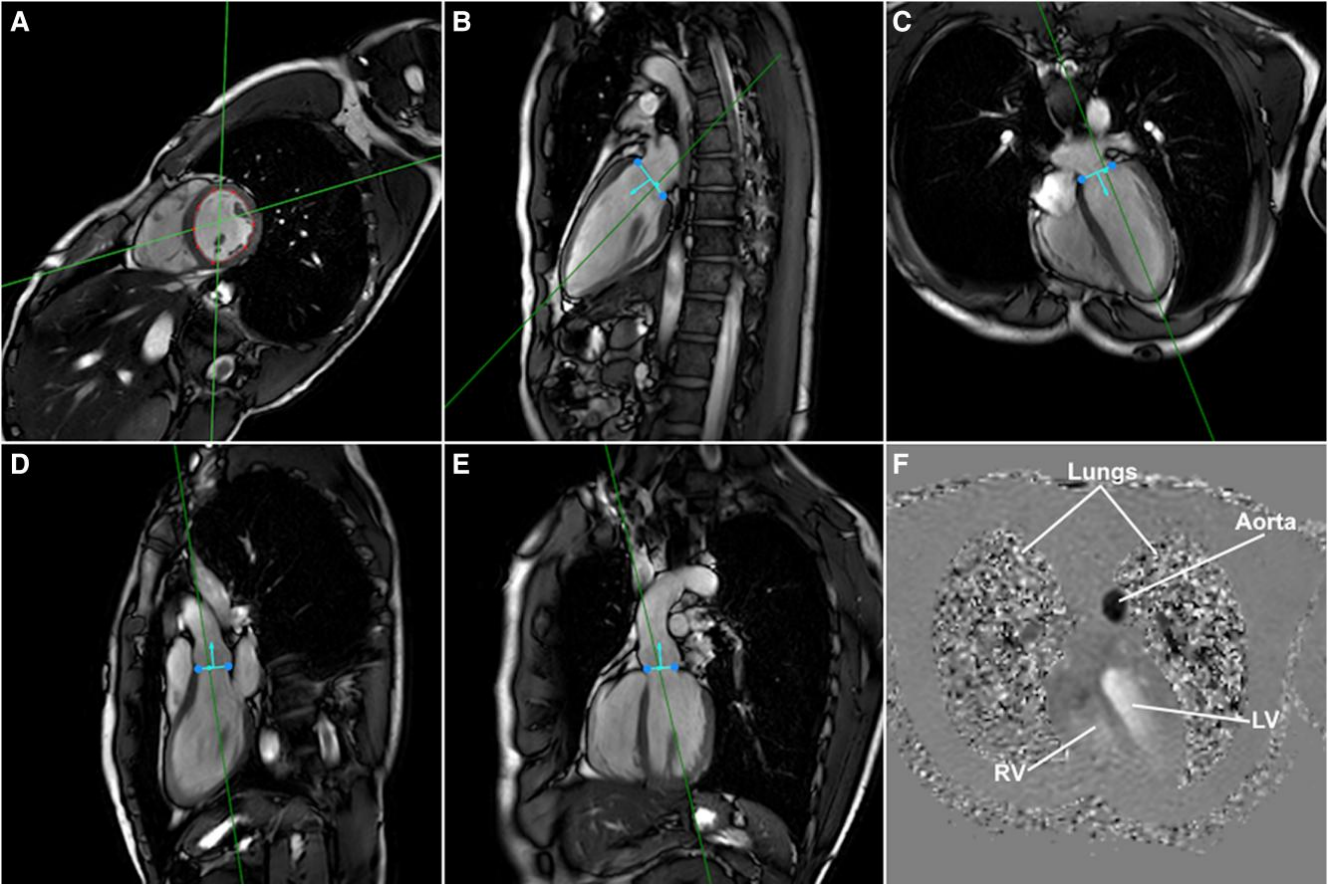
CMR scans with segmentation and valve tracking, as used to perform four-component intracardiac particle tracing. (*A–E*) LV cine segmented fast gradient echo scans of short-axis, two-chamber left, four-chamber, sagittal aortic annulus, and coronal aortic annulus, respectively. Straight lines depict location and orientation of scans that are perpendicular to the image plane. Blue lines and arrows depict mitral annulus (*B* and *C*) or aortic annulus (*D* and *E*) location and flow direction. (*F*) Phase image of 4D flow MRI with velocity encoding in feet–head direction with time point chosen during peak-systole.
